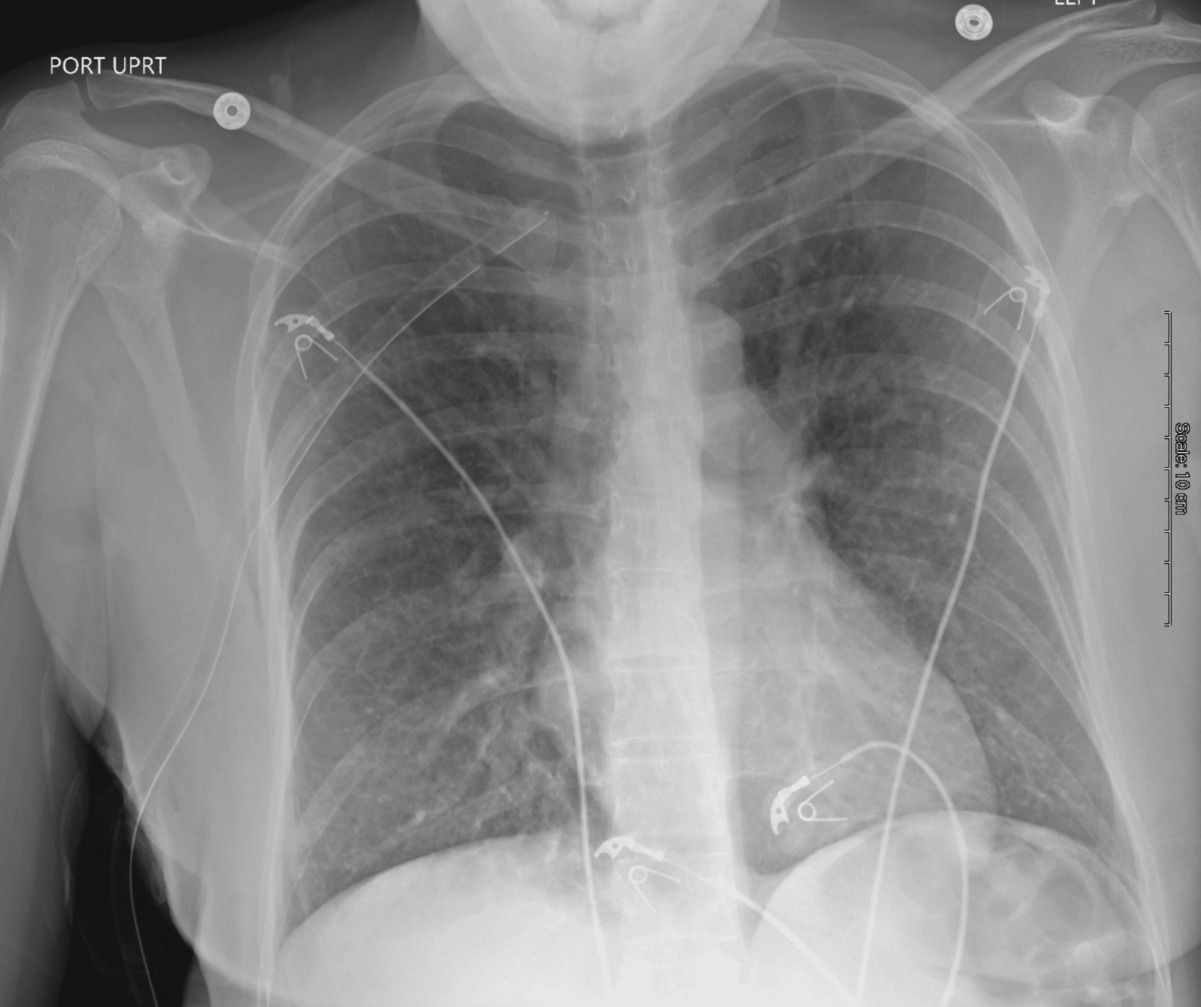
AP chest X-ray after chest tube insertion showing resolution of pneumothorax with adequate chest tube placement AP: anteroposterior
